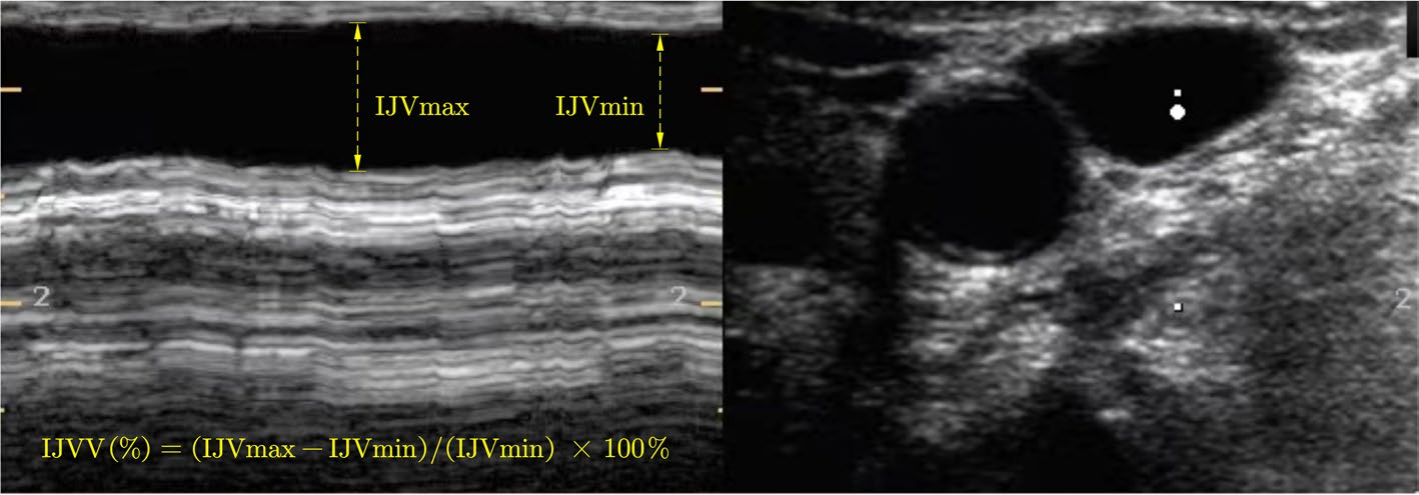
Representative ultrasound image of the IJVV. IJVV, respiratory variation in the internal jugular vein
